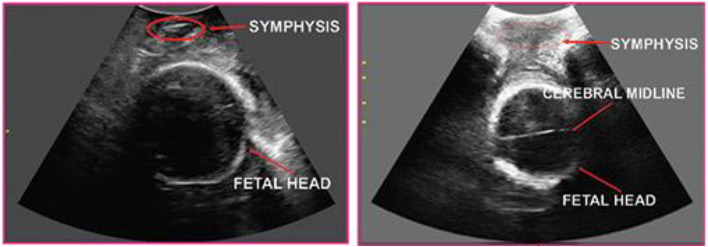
Eco view panel of Anatomical points in Ultrasound’s measurement
